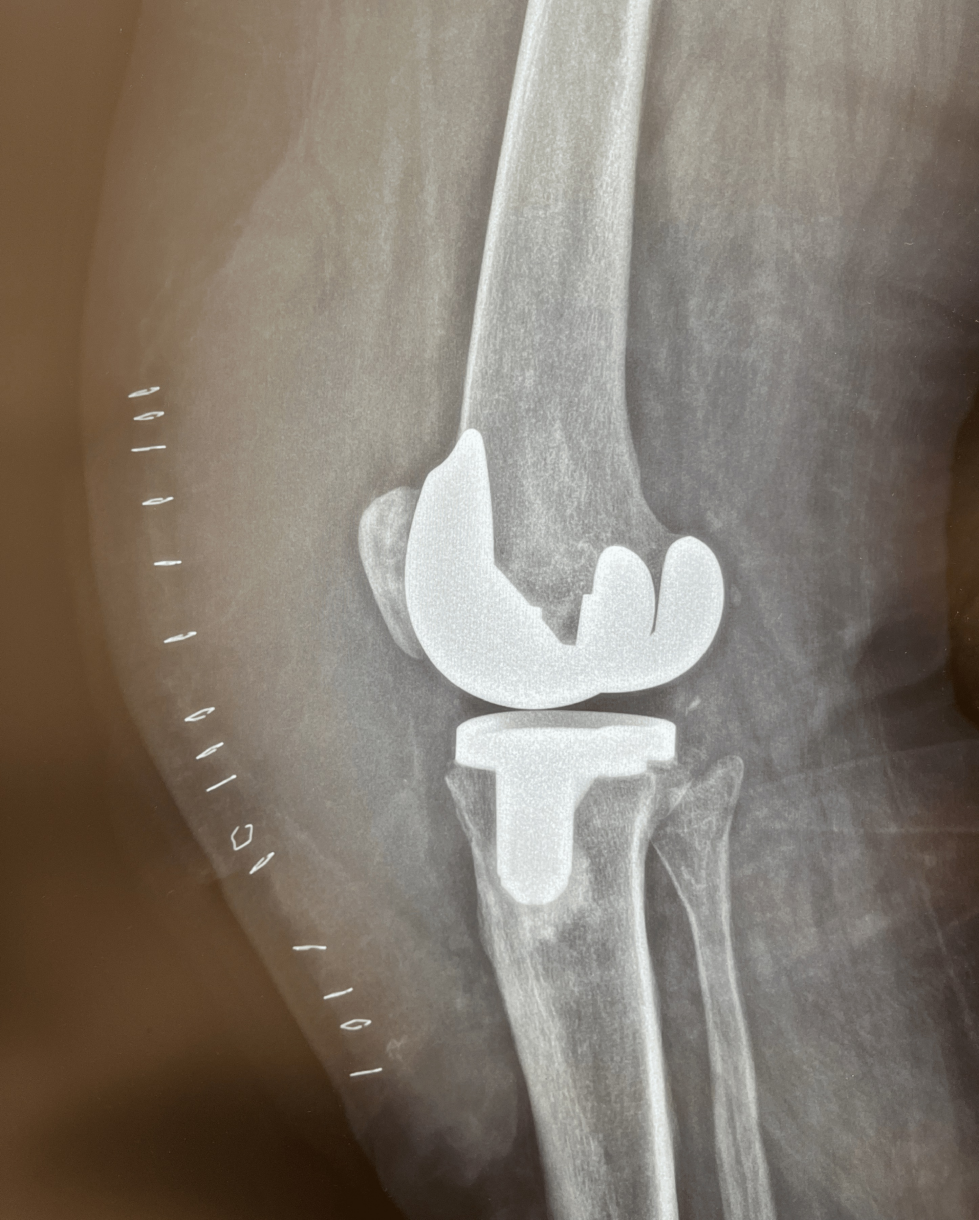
Lateral radiograph of the knee at presentation
